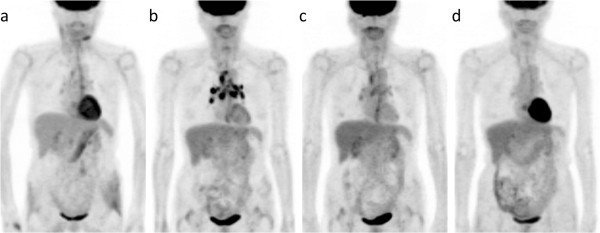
Case 2. FDG-PET/CT images that were conducted 0 (a) and 9 months (b) after the completion of antineoplastic therapy for mandibular osteosarcoma and 6 (c) and 28 months (d) after the detection of FDG-avid lesions.
